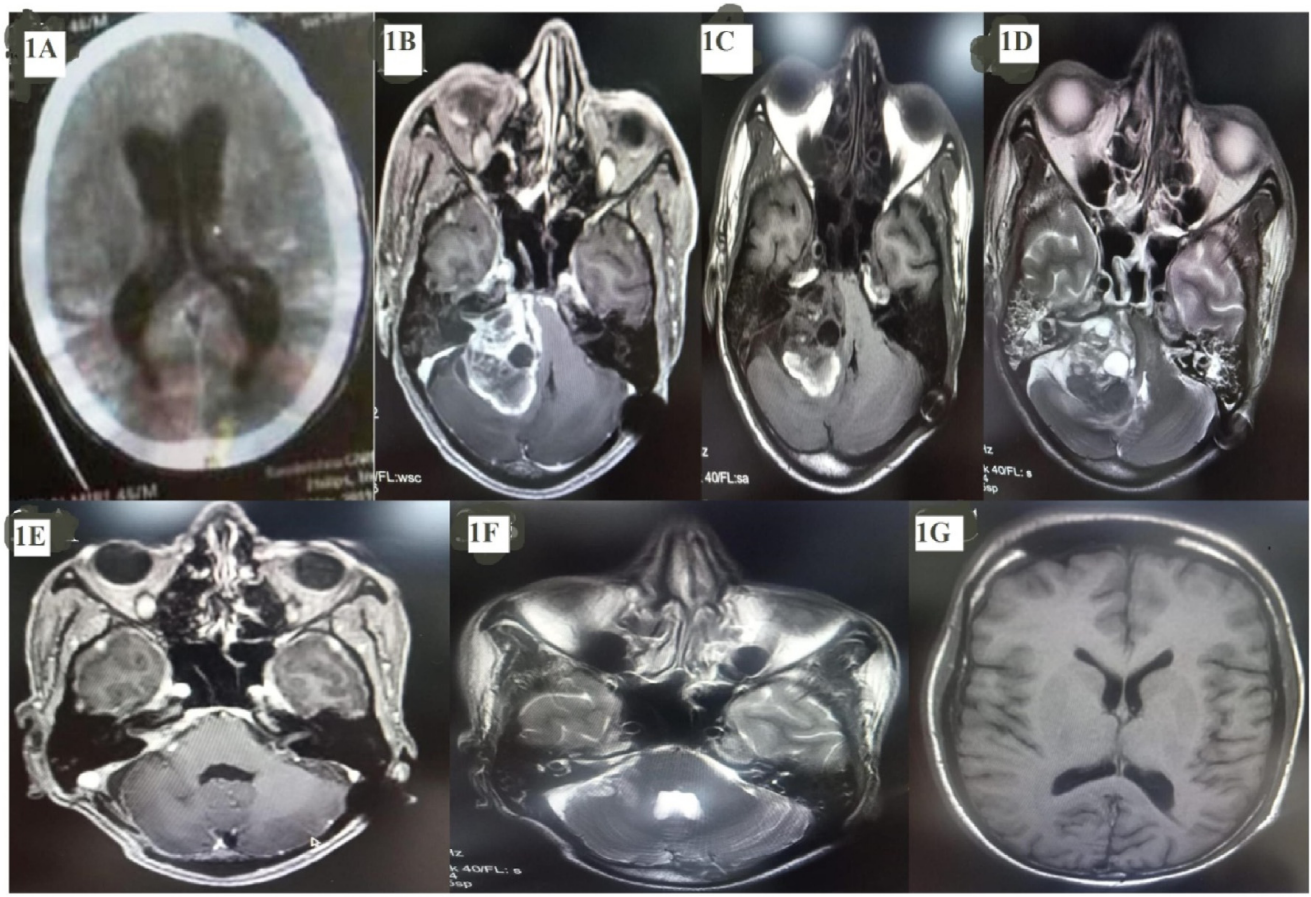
(1A) Computed tomography (CT) brain plain showed dilation of bilateral lateral ventricle suggestive of hydrocephalus at the time of admission. Fig. 1: (1B) T1 weighted with contrast MRI (Magnetic Resonance Imaging) showed 5.4 cm ​× ​3.8 cm sized enhancing solid tumor in right CP angle with extension into the right IAM. (1C) T1 weighted MRI image showed a predominant hypointense lesion in the right cp angle region extending into IAM, with hyperintensity in the periphery of lesion with severe brain stem compression. (1D) T2 weighted image revealed a hyperintense lesion in the right CP angle with hypointensity in the periphery of lesion suggestive of an early subacute phase of blood with no brainstem signal changes. Figure 1(E,F,G) showed postoperative MRI- (1E) T1 weighted with Gadolinium contrast MRI showed small residue in right IAM, in (1F) T2 weighted image showed postoperative changes seen in the right cerebellum and no brain stem compression. (1G) showed resolution of hydrocephalus.
